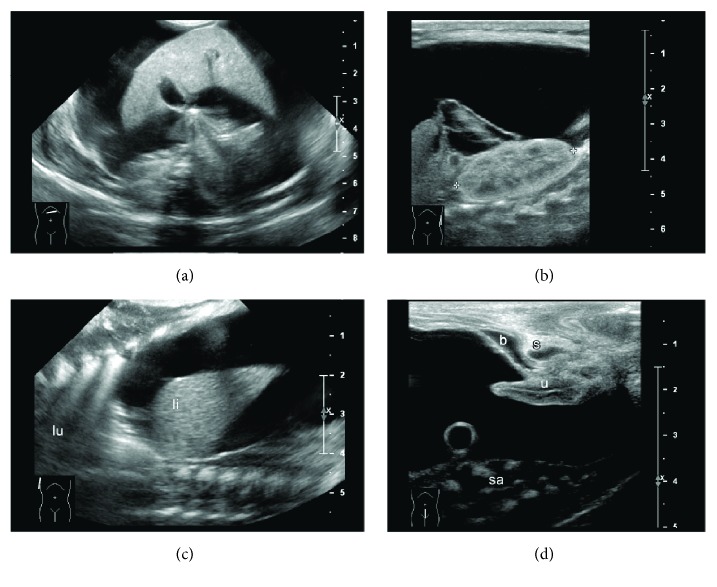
Abdominal ultrasound images showing massive ascites. (a) Transverse section through the epigastrium. (b) Longitudinal section through the left kidney that appears normal in shape and length. (c) Longitudinal section through the liver and lung (li: liver; lu: lung) showing no pleural effusion. (d) Longitudinal section through the bladder (b: bladder; u: uterus; sa: sacrum; s: symphysis), which is almost empty.
